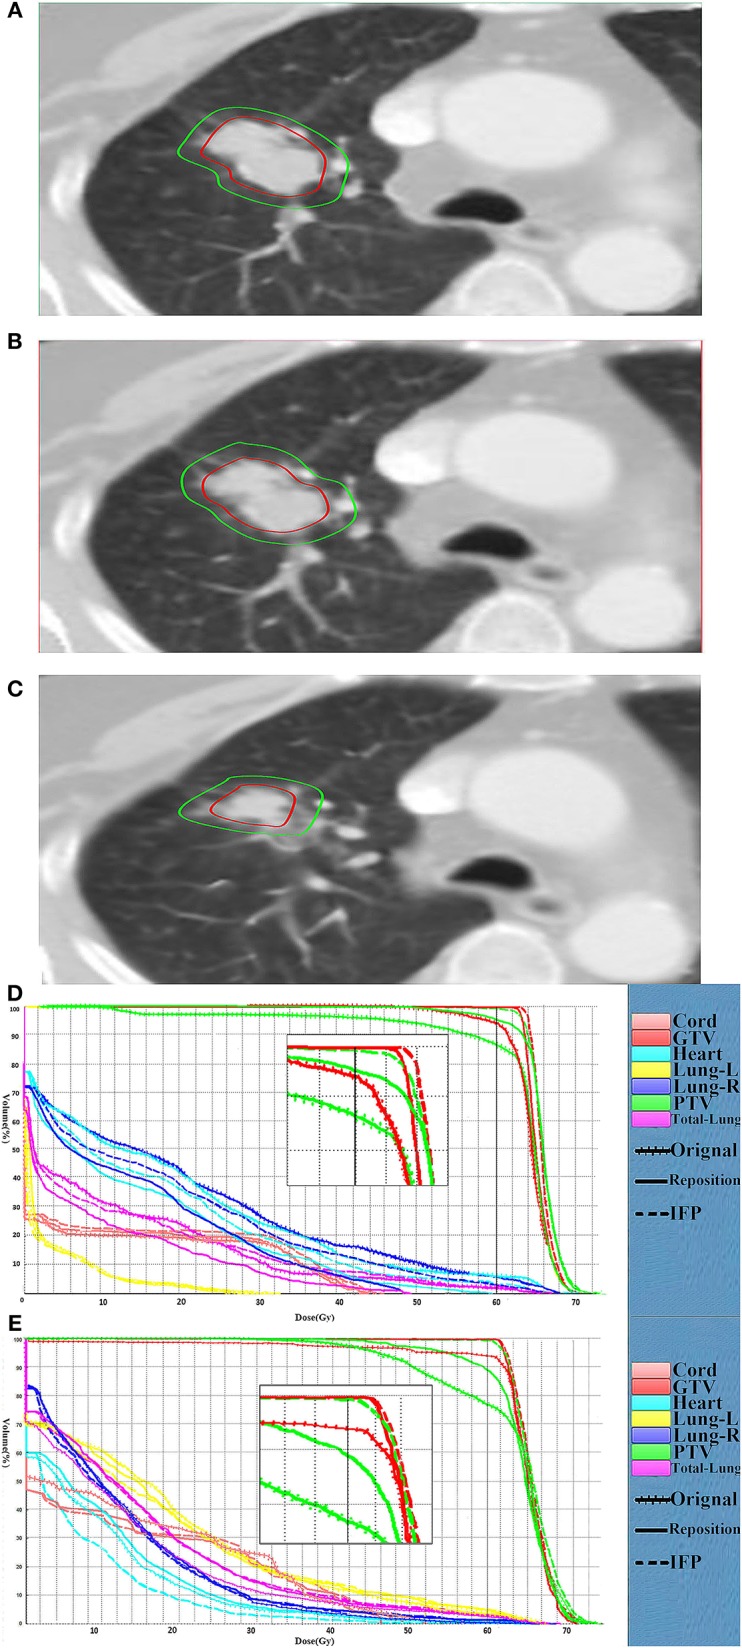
Comparison of the target volume in the representative CT slice. Comparison of the target volume in the representative CT slice obtained on the CT-plan (A), the corrected CT of fraction 6 (B) and the corrected CT (C) of fraction 16, and DVH curves comparison for the fraction 6 (D) and fraction 16 (E) for patient 1. The dotted, dashes, and solid curves represent the original, IFP and the repositioning plans, respectively.
